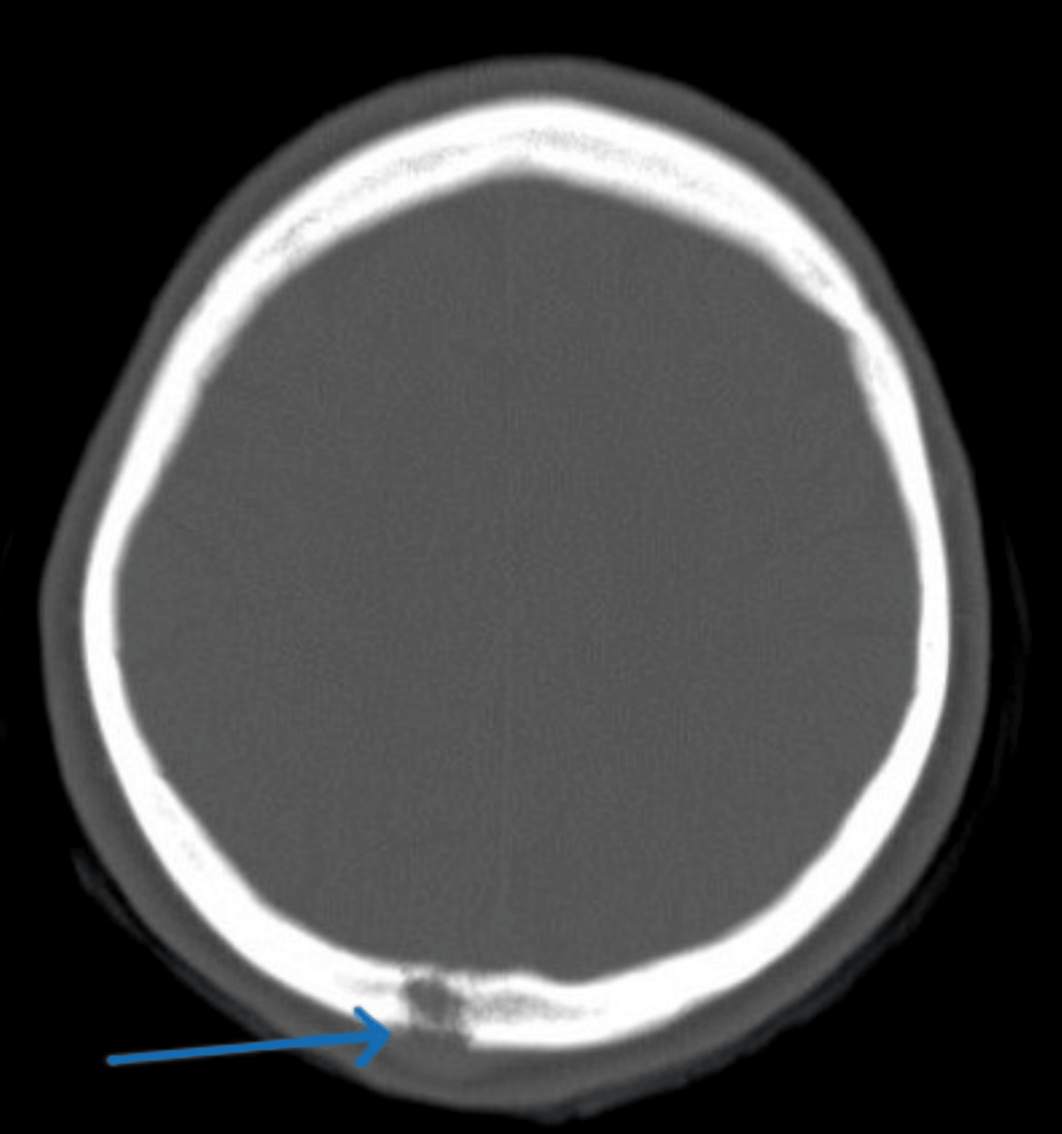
A CT scan of the brain bone window demonstrates a lytic lesion in the occipital bone.
